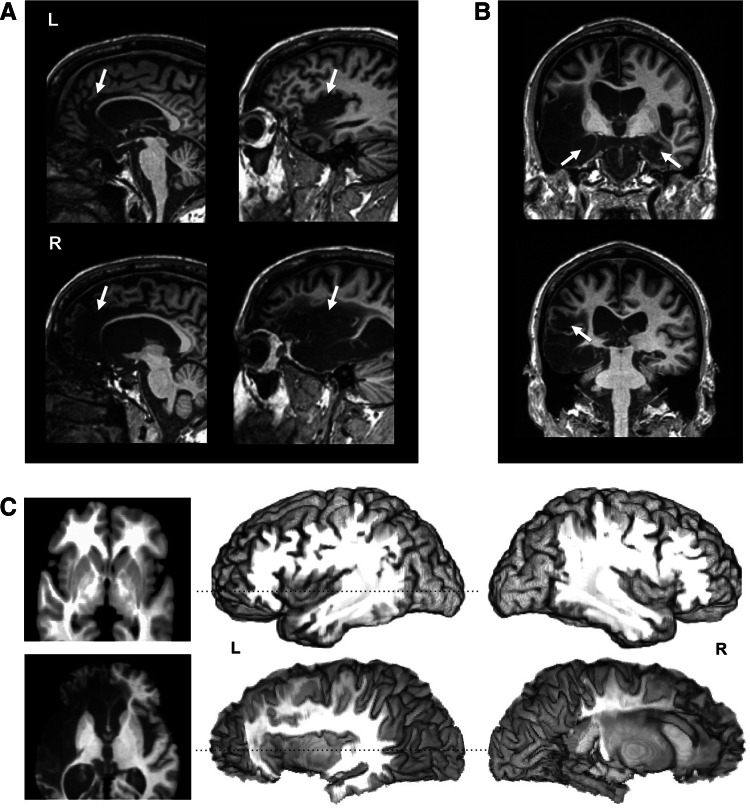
Roger’s brain. a Sagittal MRI slices showing bilateral lesions to the ACC (*leftmost*
